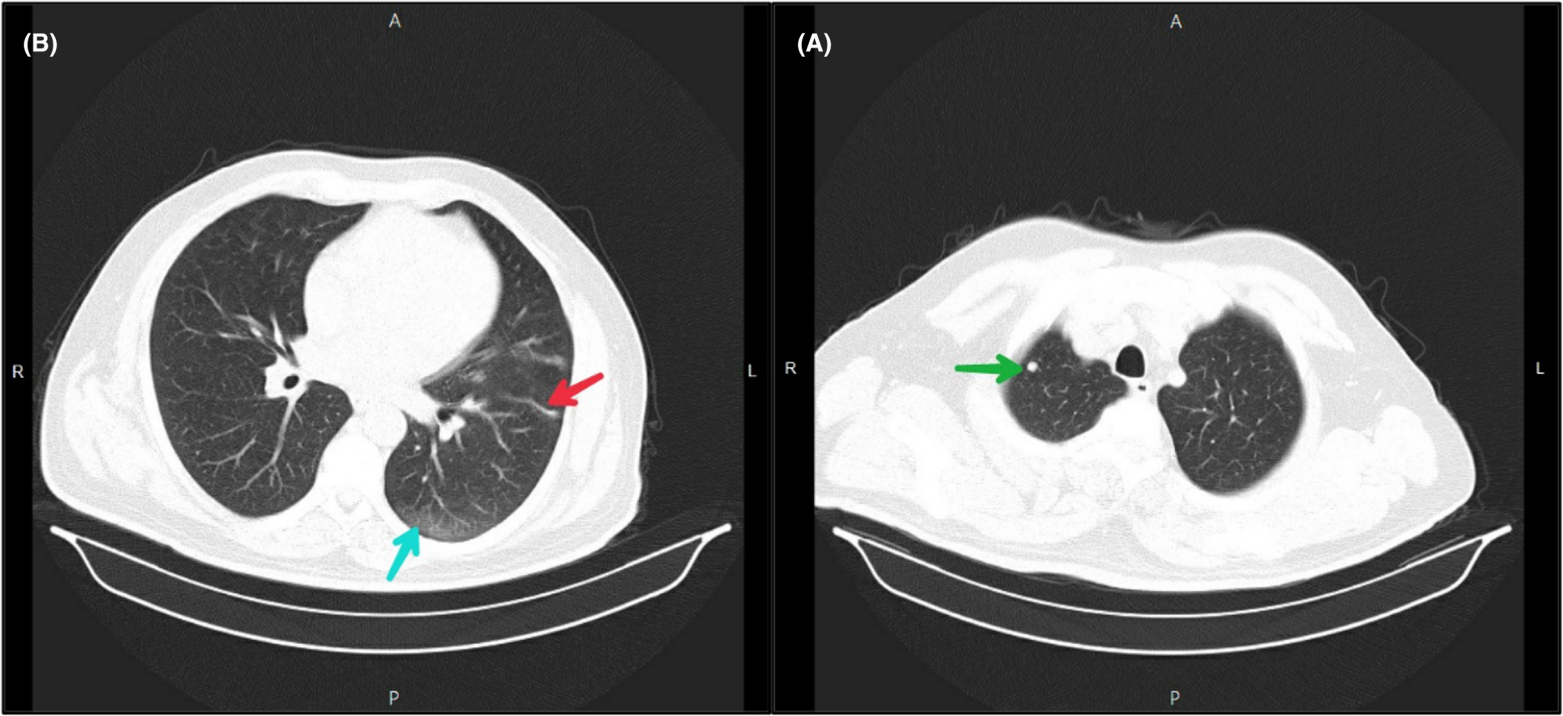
Parenchymal view of the spiral chest CT scan at admission. (A) Nodule with sharp edges in RUL (green arrow); (B) Peripheral ground glass opacity (blue arrow) and atelectatic band in the left lung parenchyma (red arrow)
